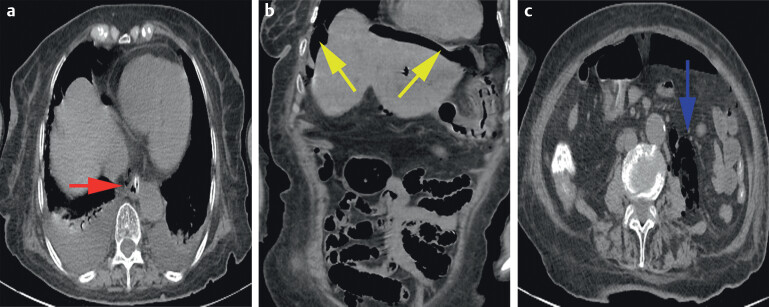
Follow-up computed tomography (CT) on day 3 demonstrating
